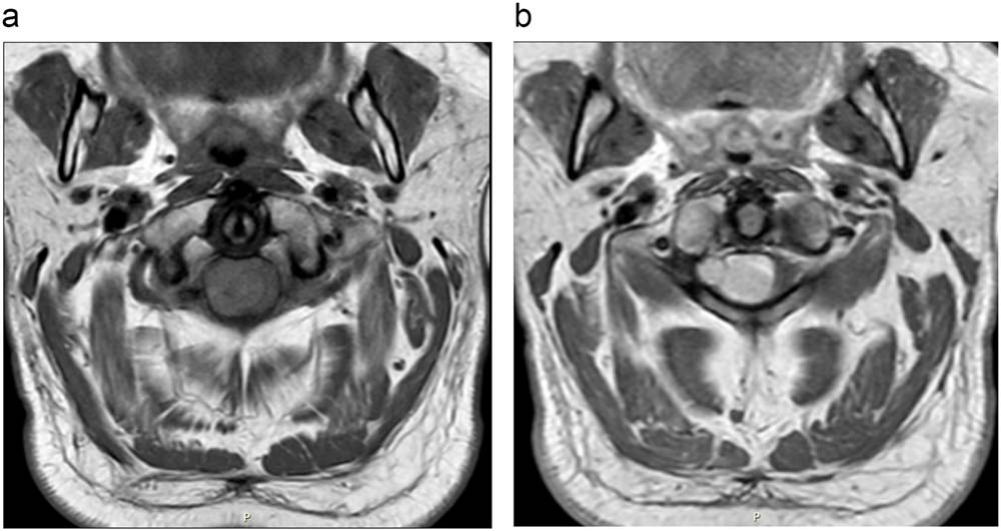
(a) Pre-operative axial MRI cervical spine T1-weighted image without contrast (across the C1 level). (b) Pre-operative axial MRI cervical spine T1-weighted image with contrast (across the C1 level).
